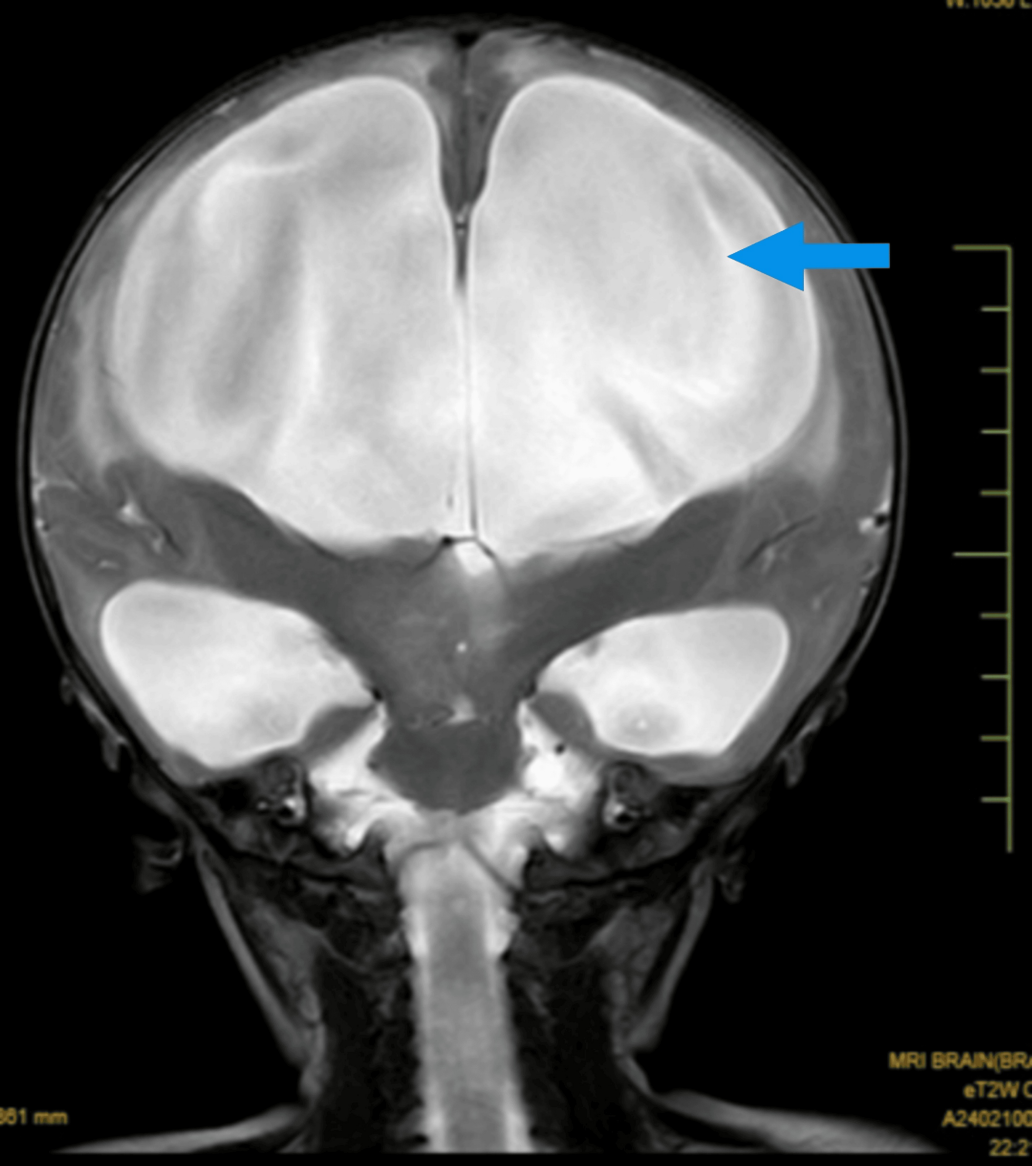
MRI brain showing hydrocephalus with subdural hygroma and pneumocephalus The arrow indicates dilatation of bilateral ventricles and diffuse involvement of the brain parenchyma along with the presence of subdural hygroma and pneumocephalus.
